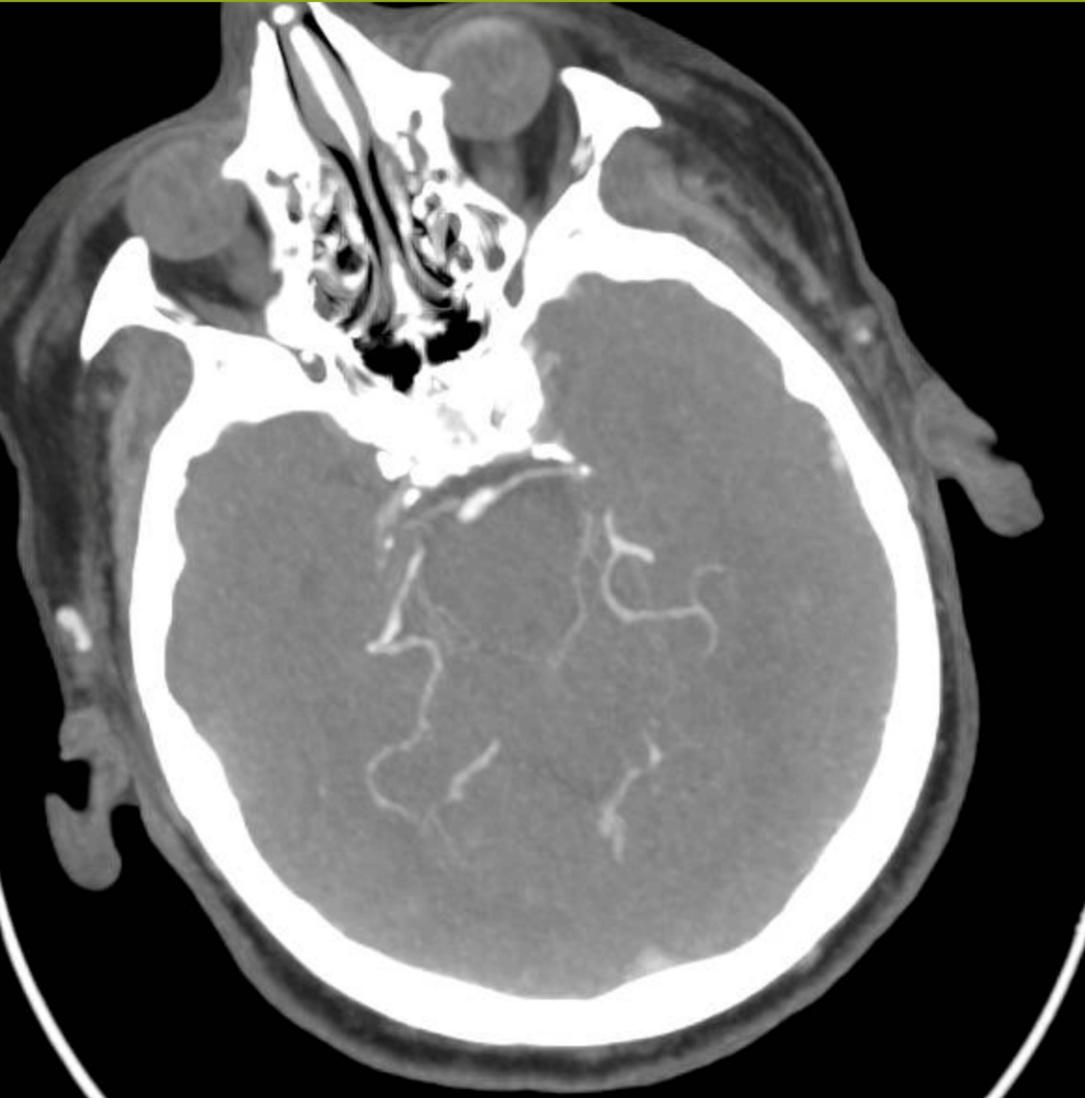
CT angiogram (axial view) showing narrowing of the P2 segment of the left PCA. PCA, posterior cerebral artery
